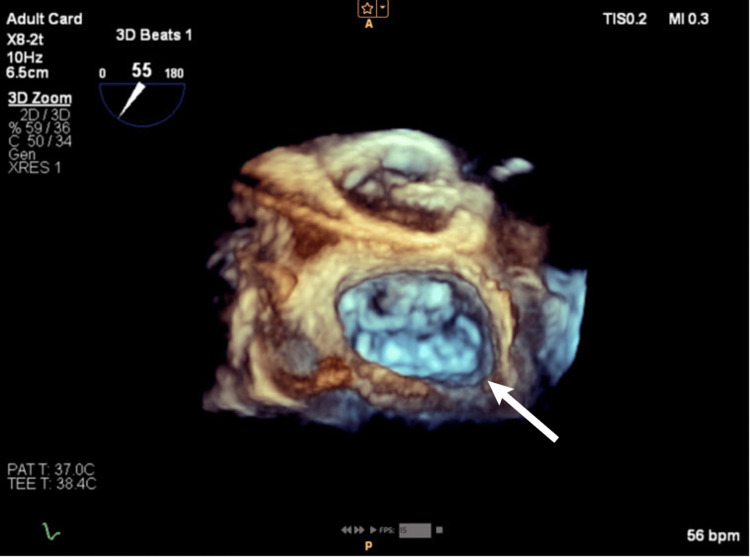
Real-time 3D TEE image of ASD II from the RA perspective. TEE: transesophageal echocardiography; RA: right atrium; ASD II: secundum atrial septal defect
